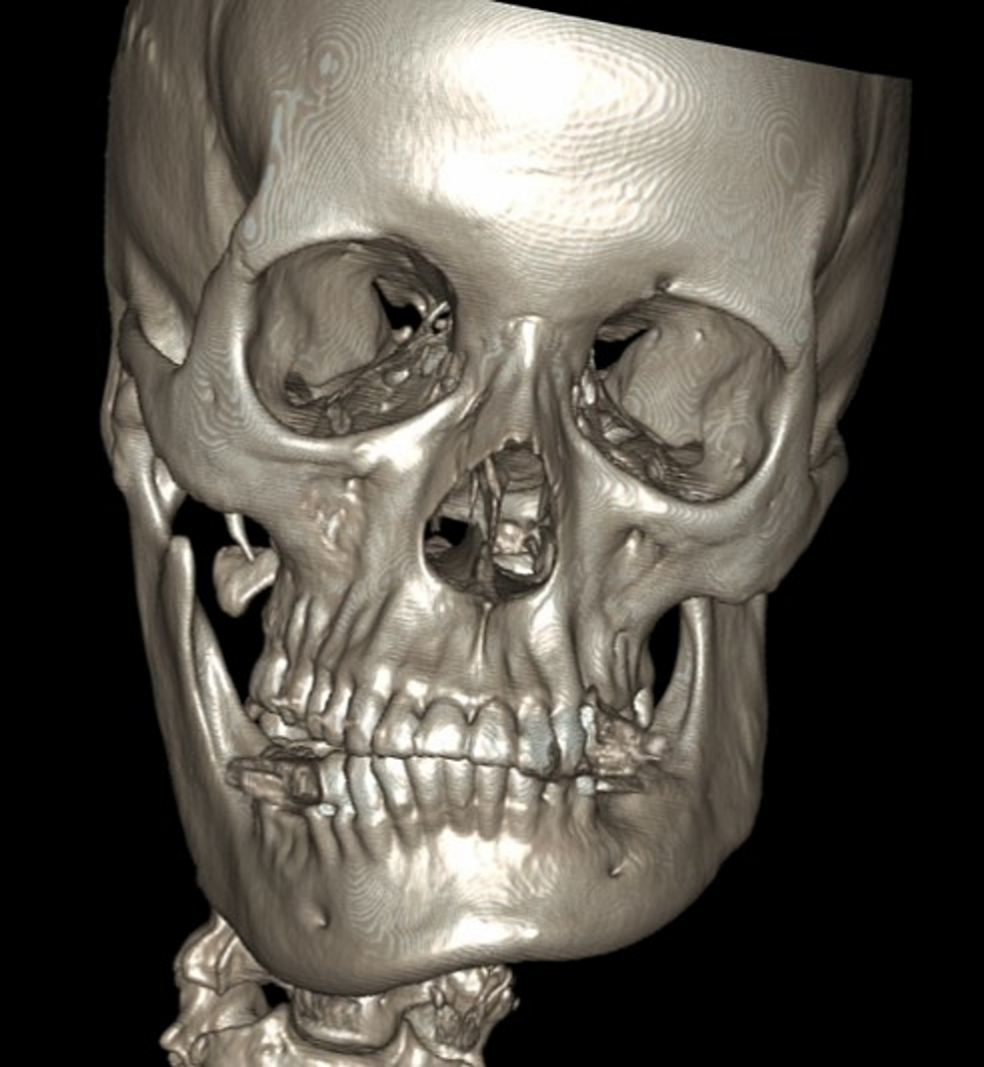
3D volume-rendering CT of the face showing asymmetry of the mandible. 3D: three dimensional; CT: computed tomography
